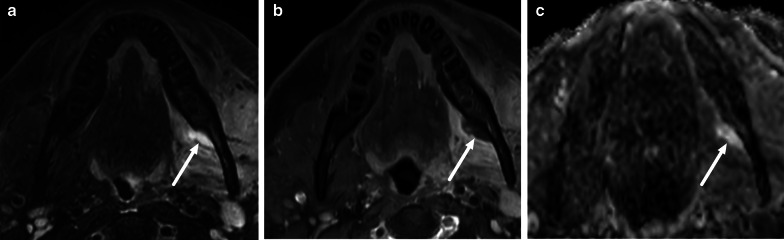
Sterile subperiosteal collection (arrows) after oral surgery on axial fat-suppressed axial T2-weighted (a) and post-contrast T1-weighted (b) images and ADC (c). High ADC values suggest sterile fluid rather than pus
